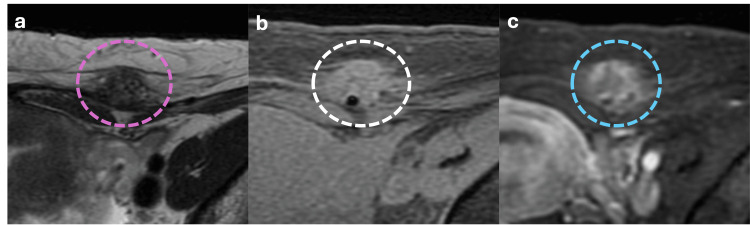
Axial abdominal MRI showing abdominal wall endometriosis deposits (a, b, c) Axial MRI showing a T2 hypointense and T1 slightly hyperintense lesion in the anterior abdominal wall (pink and white dotted circles, respectively), with delayed contrast enhancement after contrast medium administration (blue dotted circle); these findings in the context of a young female with history of cesarean section are highly suspicious for endometriosis deposits. The figure was created by the authors.
